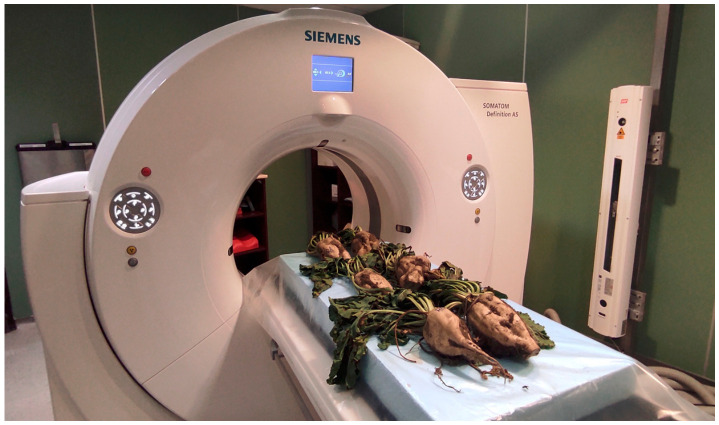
The analysed sugar beet samples in the Siemens CT scanner. The acquisition parameters with a 100 kV tube voltage, with an X-ray dose of 250 mAs, spiral data collection mode with a pitch of 0.4, slice thickness of 0.6 mm, field of view (FoV) of 102 mm, and convolution kernel were I50f. The data collection takes 60–90 s, so the scan did not exceed 10 min for the sugar beet samples analysed. The total number of reconstructed CT images ranges from approximately 1000 to 7000, depending on sample size and resolution.
